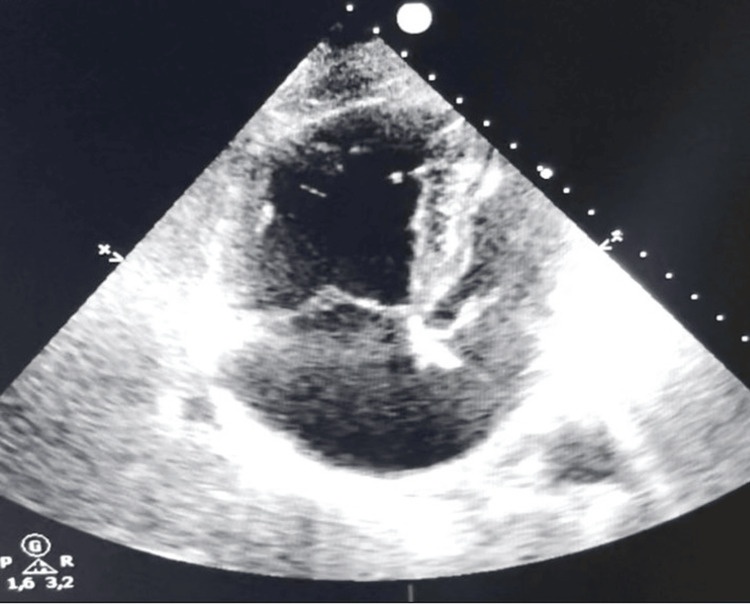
Transthoracic echocardiography, apical four-chamber view on day 15 showing complete resolution of the right atrial thrombus
